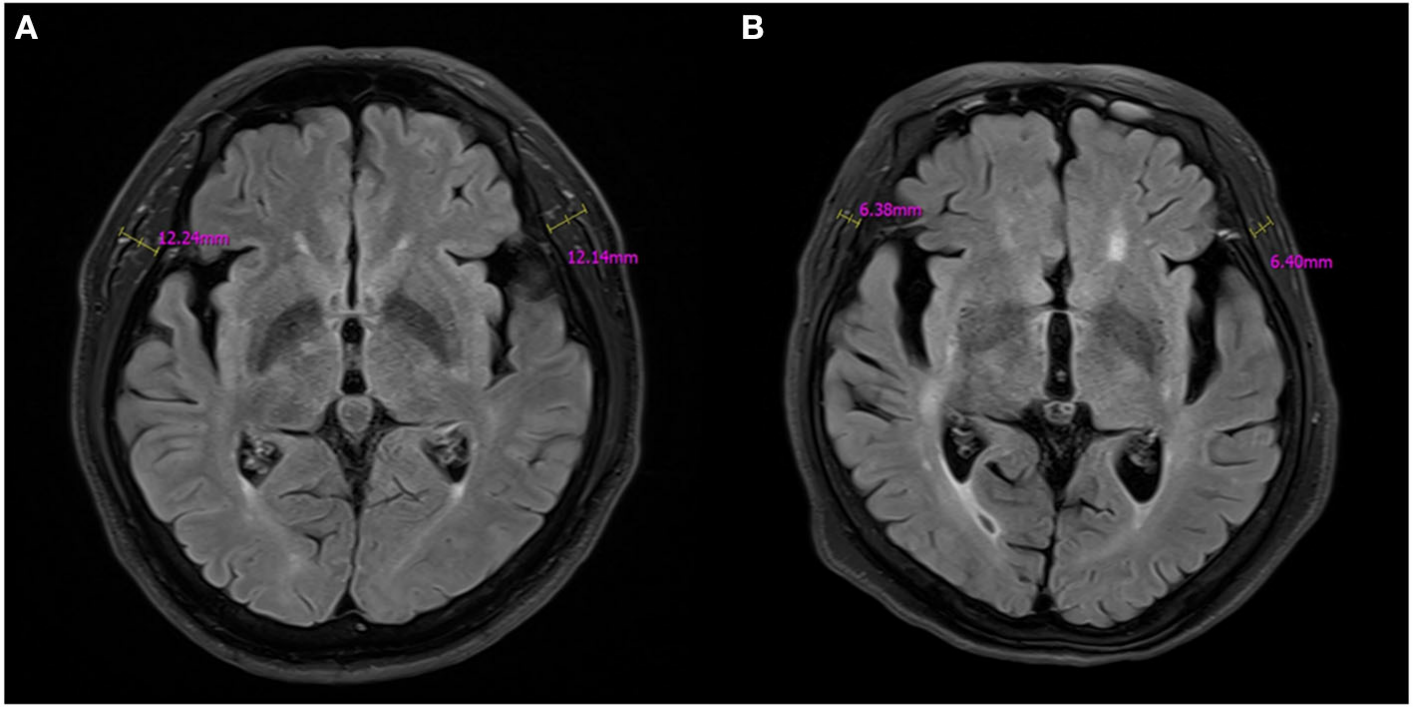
Examples of TMT measurements on MR FLAIR images. (A) A 67-year-old male patient with left basal ganglia infarction, (B) A 78-year-old female patient with left pontine infarction.
